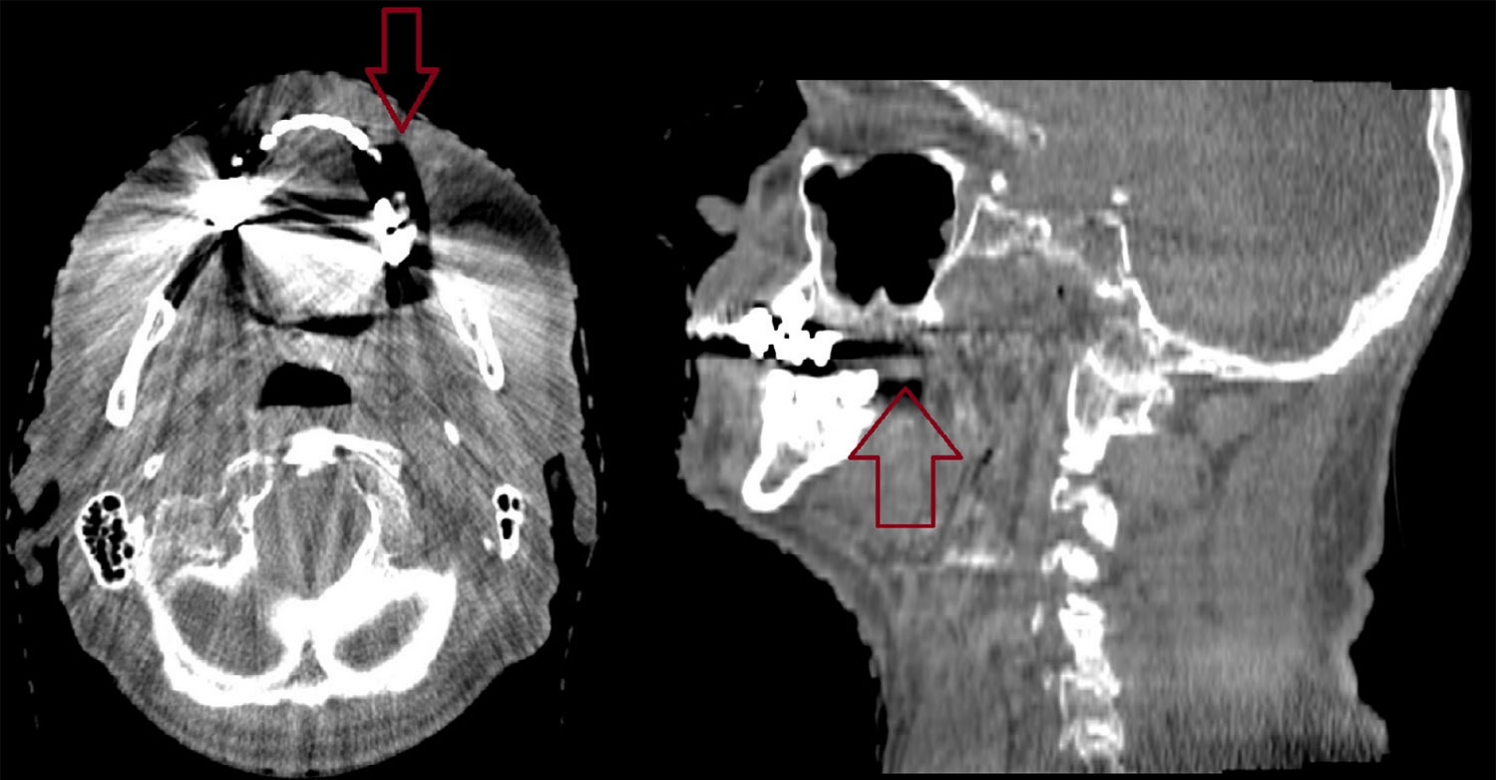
The locations of the amalgam dental fillings and the films (red arrow) on axial and sagittal CT slice.
